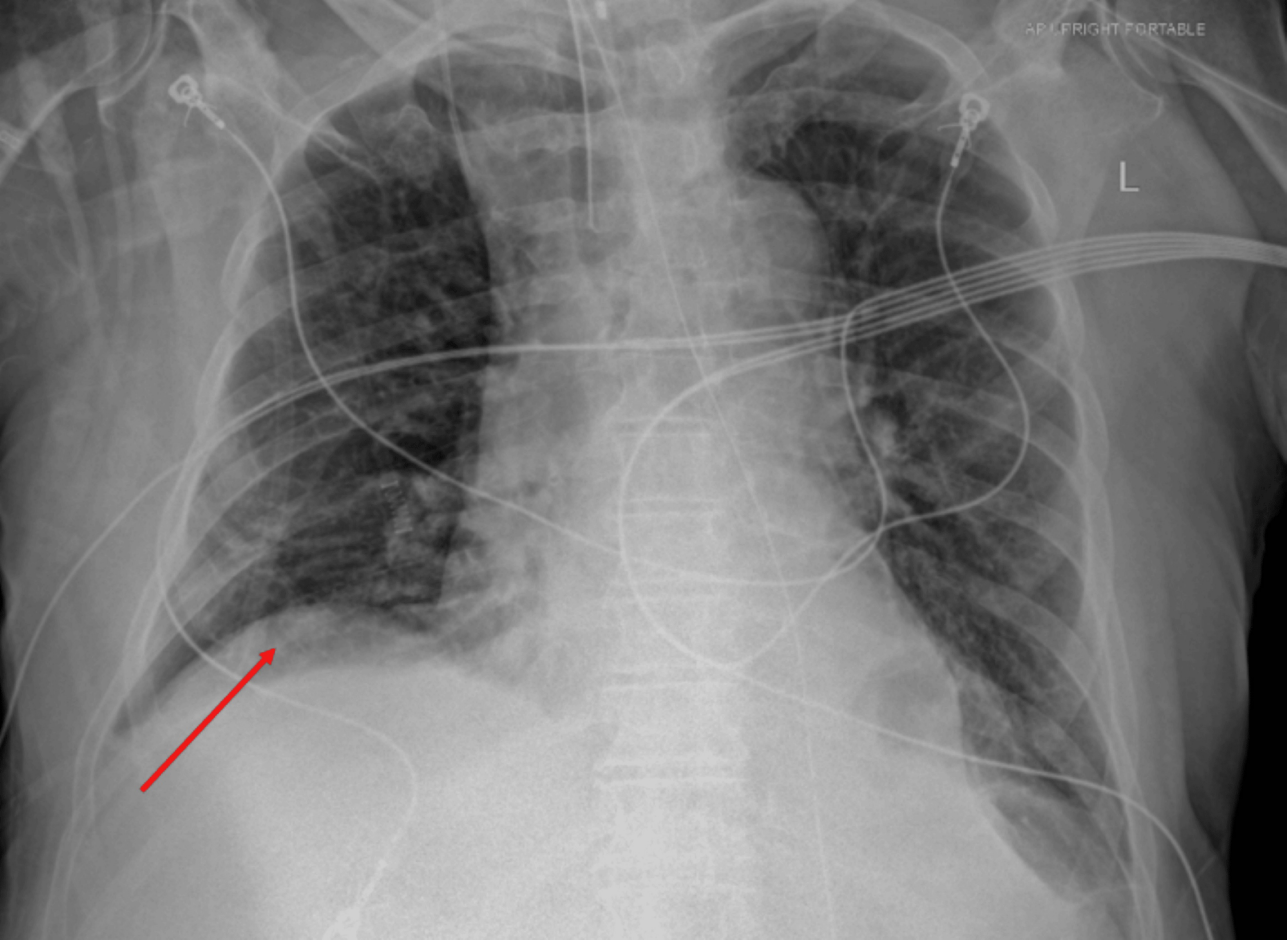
Portable chest radiograph demonstrating elevation of the right hemidiaphragm with adjacent basilar opacities (arrow) consistent with atelectasis or pneumonia
